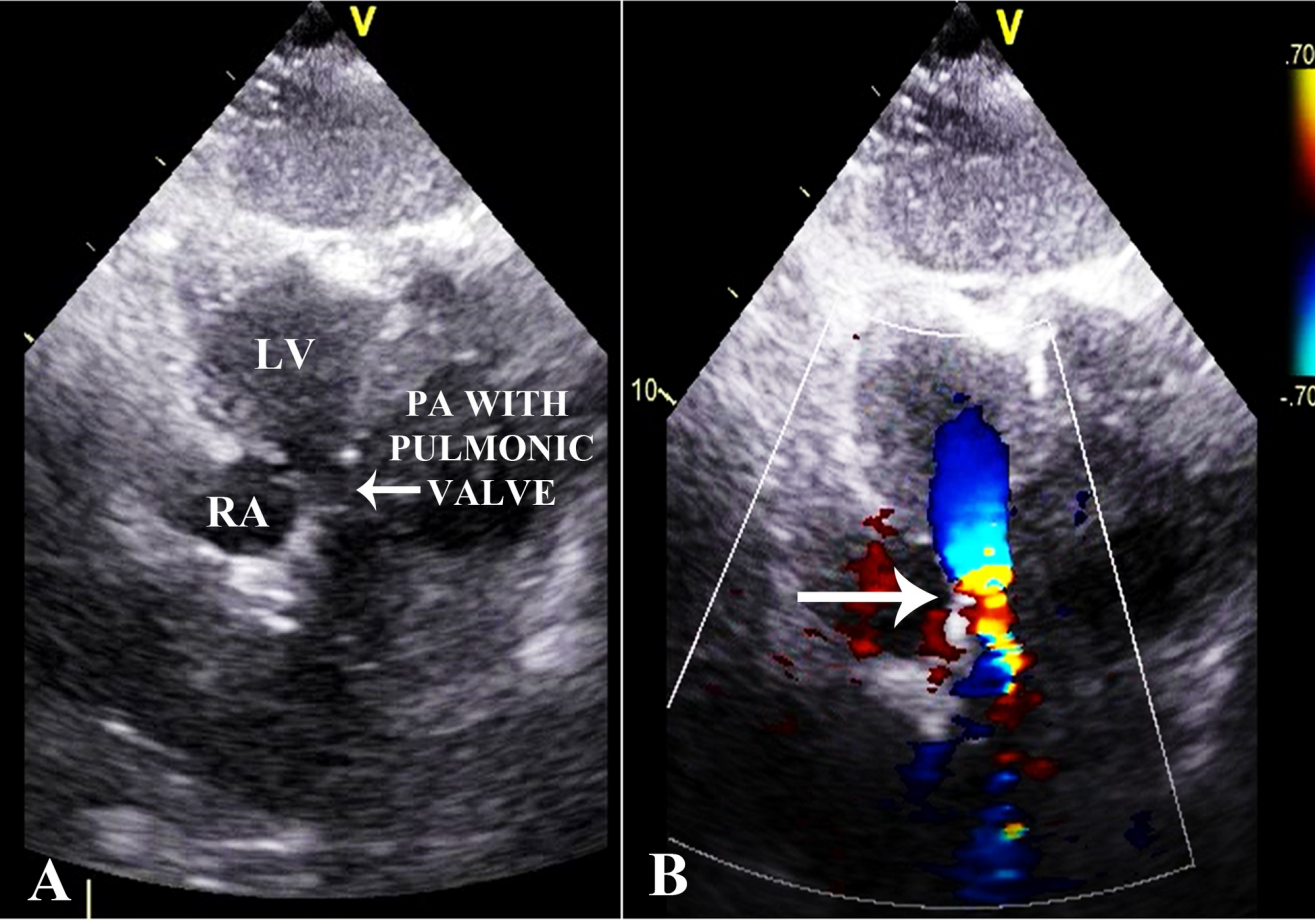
Echocardiogram. (A) Modified view showing pulmonary artery (PA) along with pulmonic valve arising from the left ventricle (LV). (B) Modified view with color Doppler applied at the level of the pulmonic valve. Forward turbulence can be seen denoting stenosis at the level of the pulmonic valve (arrow).
